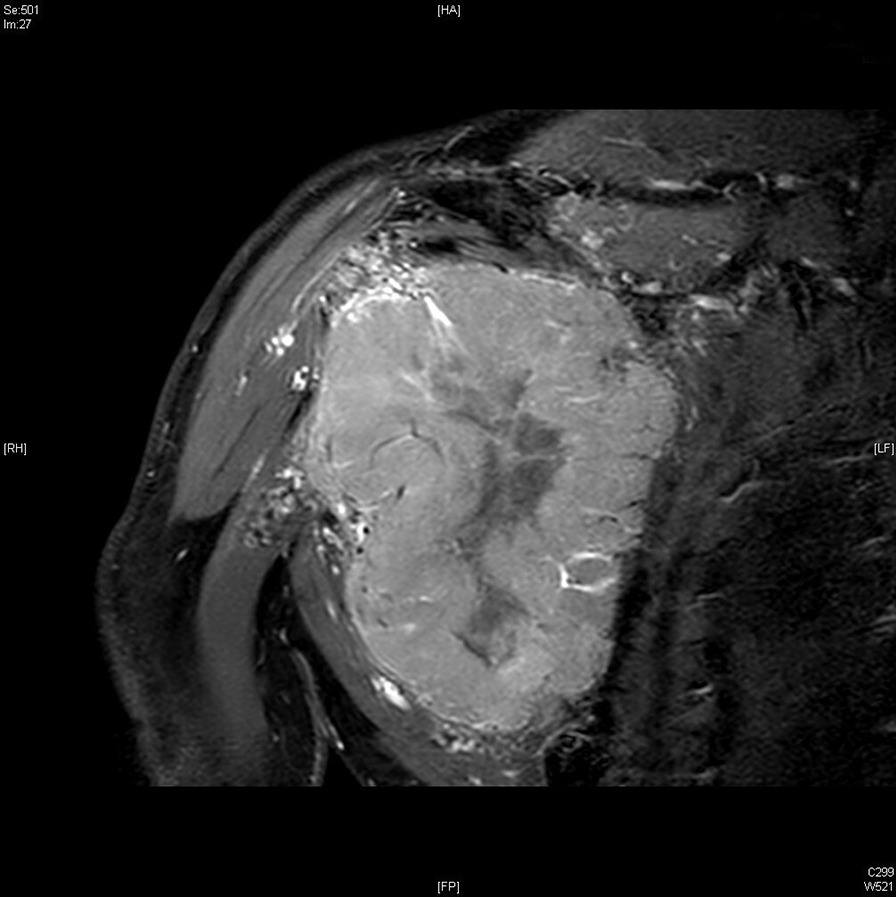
Magnetic resonance imaging of the right scapular mass
